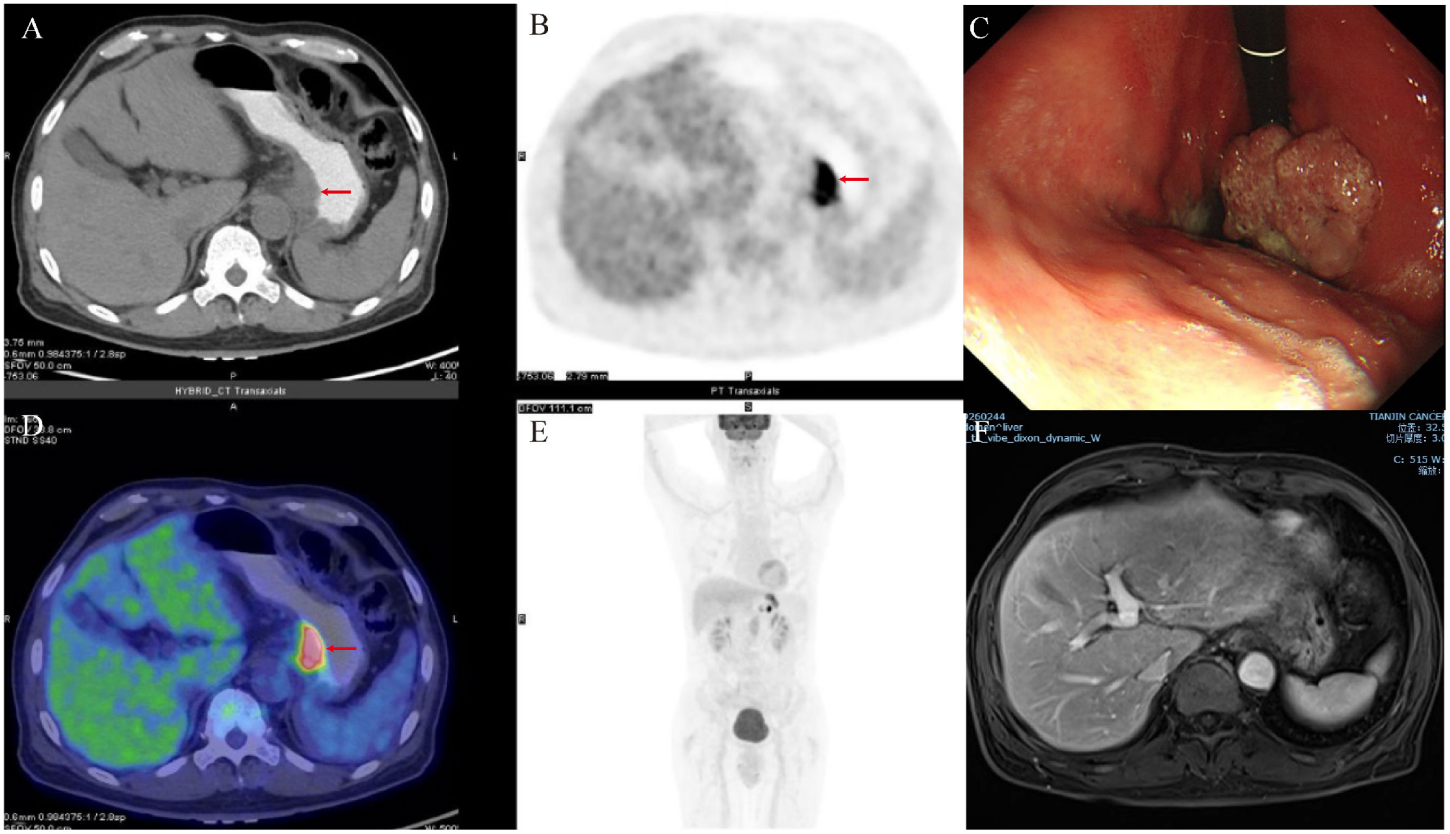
Examinations of the patient after comprehensive treatment. (A, B, D, E) Gastric tumors shrank, and liver metastases disappeared on PET−CT after neoadjuvant therapy. (C) The tumor volume showed reduction during gastroscopy. (F) MRI revealed the absence of liver metastases.
